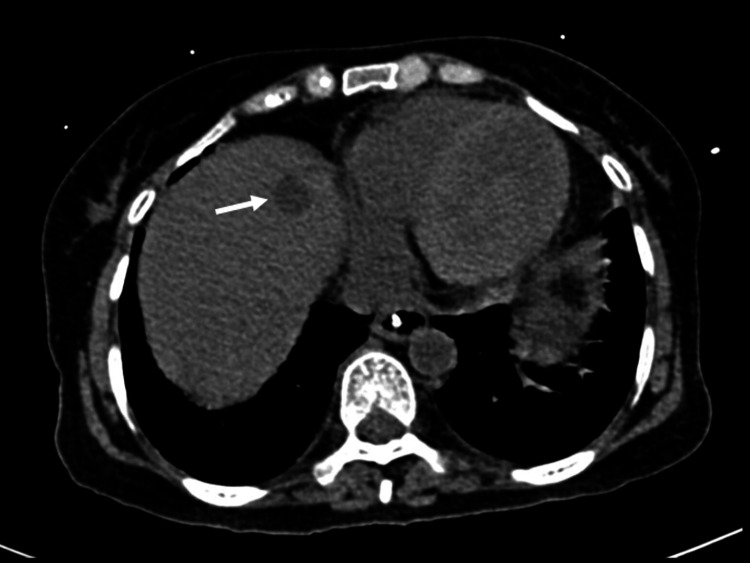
Contrast-enhanced CT (CECT) scan of the abdomen showing liver metastasis
